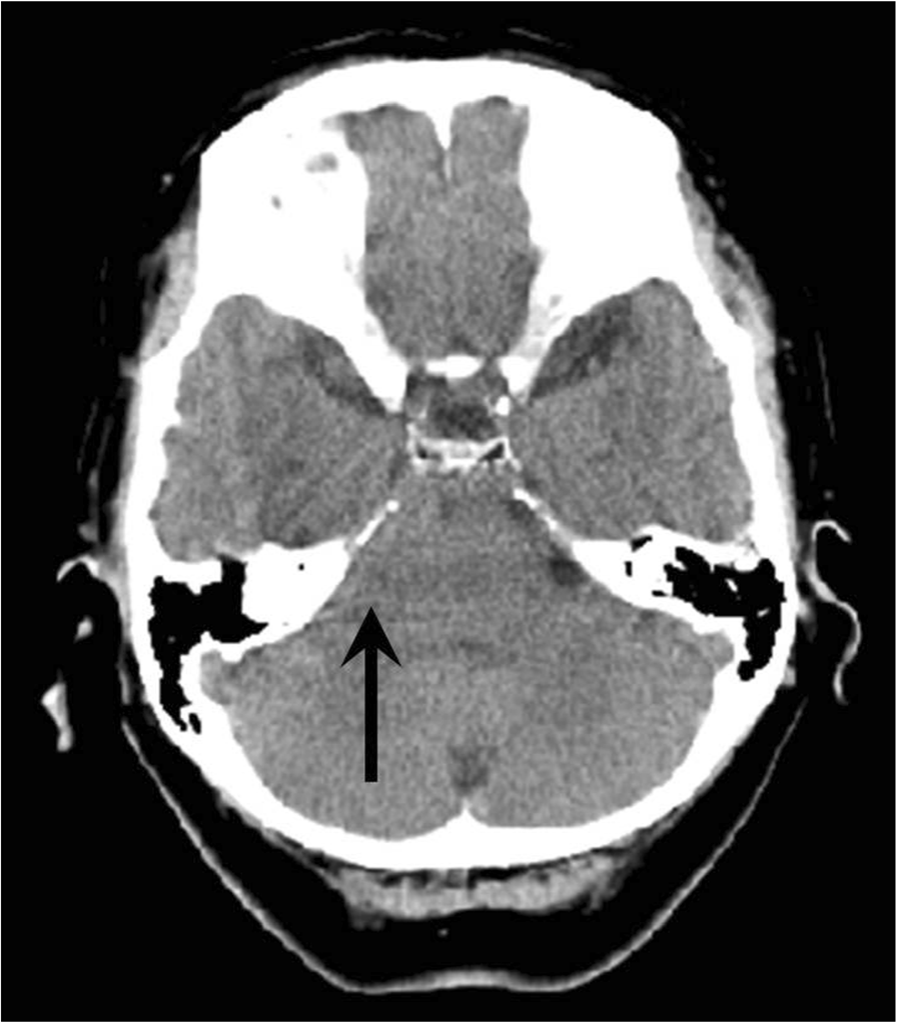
Post-surgical non-contrast computed tomography of the brain showing no intracranial hemorrhage. Subsequent image reading demonstrated the disappearance of the cerebrospinal fluid cavity at the right cerebellopontine angle (arrow)
